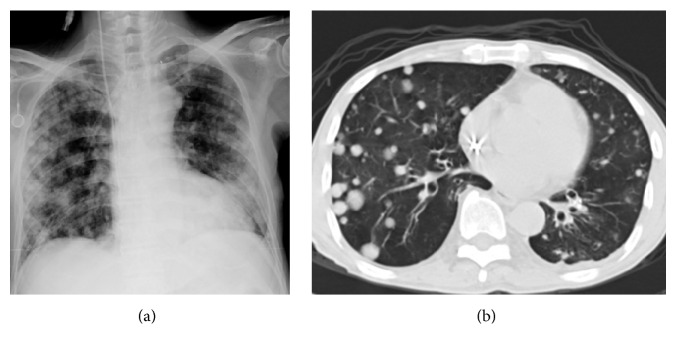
Peripheral parenchyma pulmonary lesions. The patient is a 58-year-old man with bladder urothelial carcinoma who underwent chemotherapy. He felt dyspnea. A chest X-ray (a) shows multiple lung metastases. A CT scan with a lung window (b) shows several nodules located in the inferior pulmonary vein with multiple lung parenchymal metastases.
